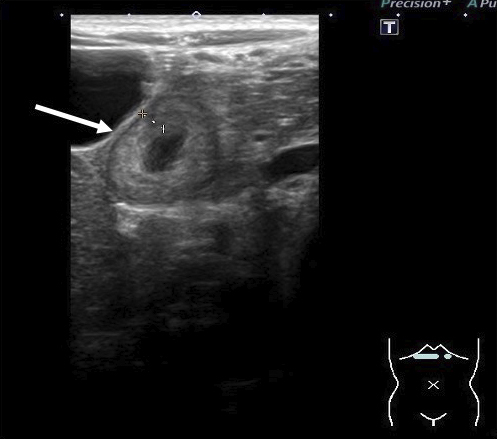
Abdominal ultrasound. The white arrow indicates wall thickening (4.1 mm) of the duodenum.
